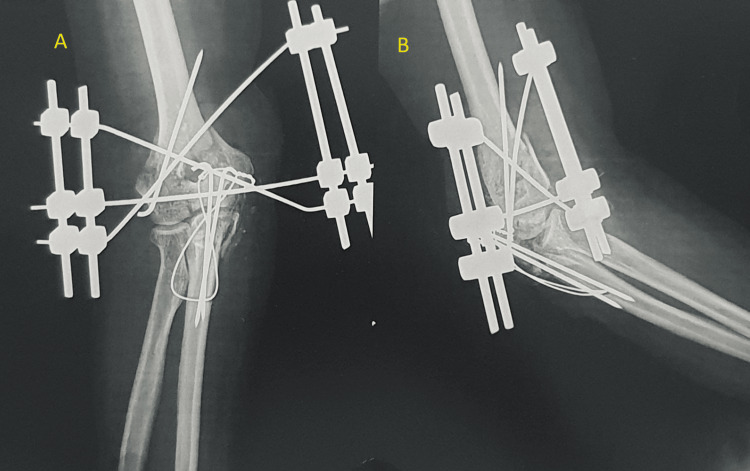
Post-operative radiograph of the right elbow with an external fixator in situ: (A) anteroposterior view and (B) lateral view
